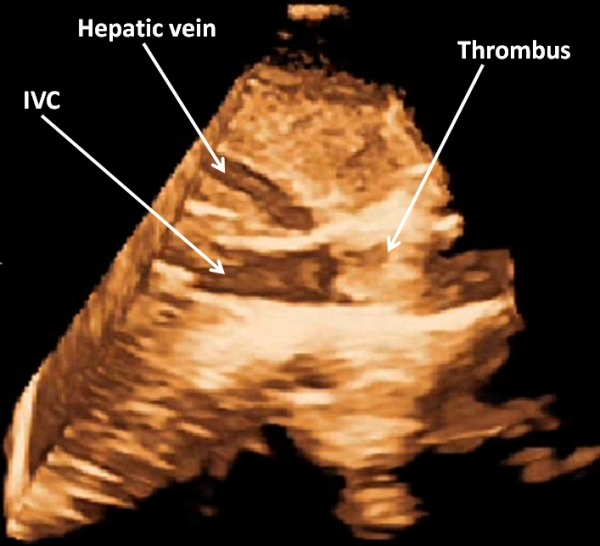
Echocardiogram showing thrombus in the inferior vena cava
